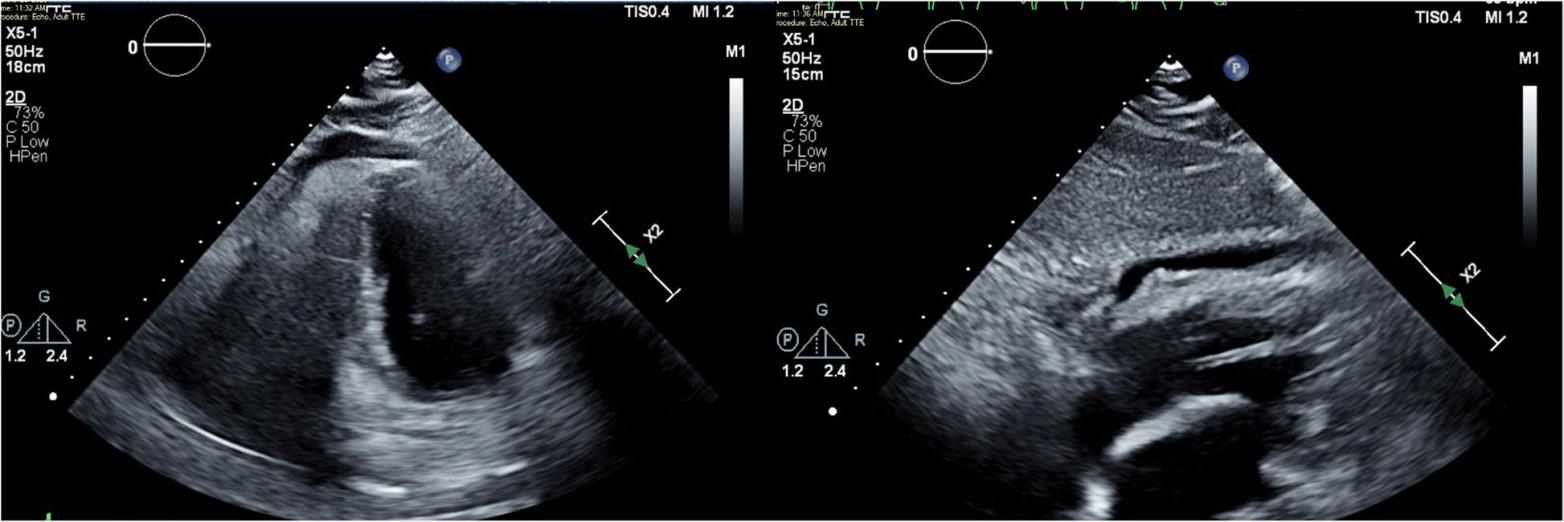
Echocardiogram with small to moderate pericardial effusion with organized material
